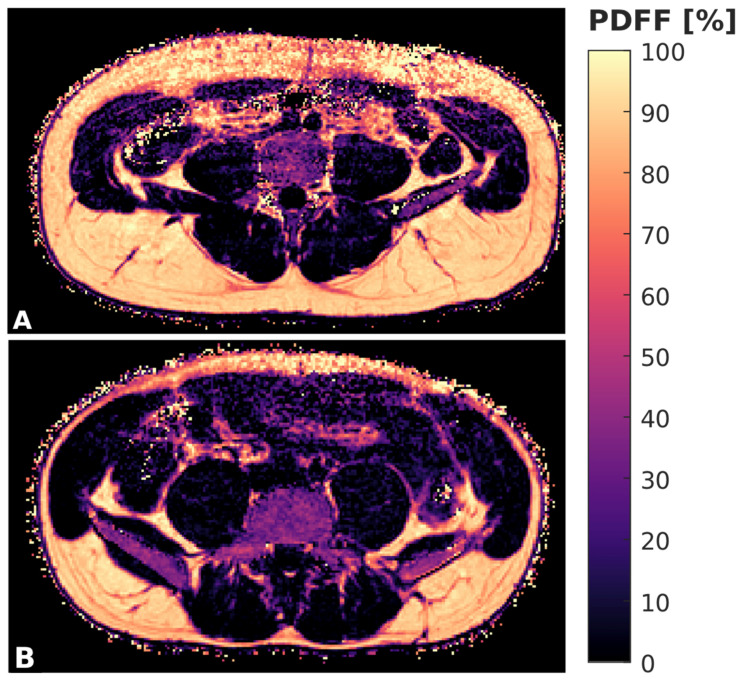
Color-coded, axially acquired PDFF maps derived from CSE-MRI of a 33 year old male (A) and 27 year old female (B) subject.
